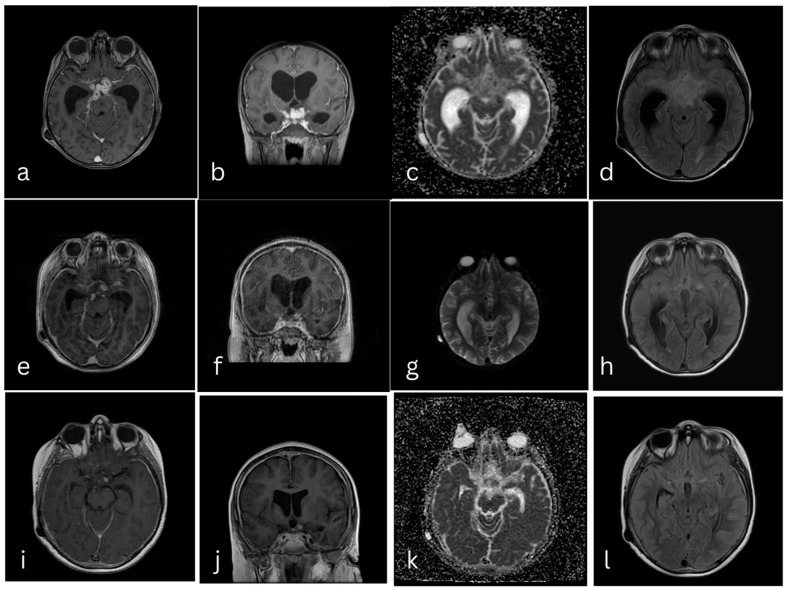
Brain MRI, T1 axial with contrast (a), T1 coronal with contrast (b), ADC axial (c), and FLAIR axial (d) after 4 months after admission, showing nodular and thickened leptomeningeal enhancement in the suprasellar region. Brain MRI 7 months after admission, T1 axial with contrast (e), T1 coronal with contrast (f), ADC axial (g), and FLAIR axial (h), showing gliosis in the nucleocapsular region. Brain MRI 12 months after admission, T1 axial with contrast (i), T1 coronal with contrast (j), ADC axial (k), and FLAIR axial (l), showing a left nodular enhancement in the suprasellar region and right hippocampal volumetric reduction.
